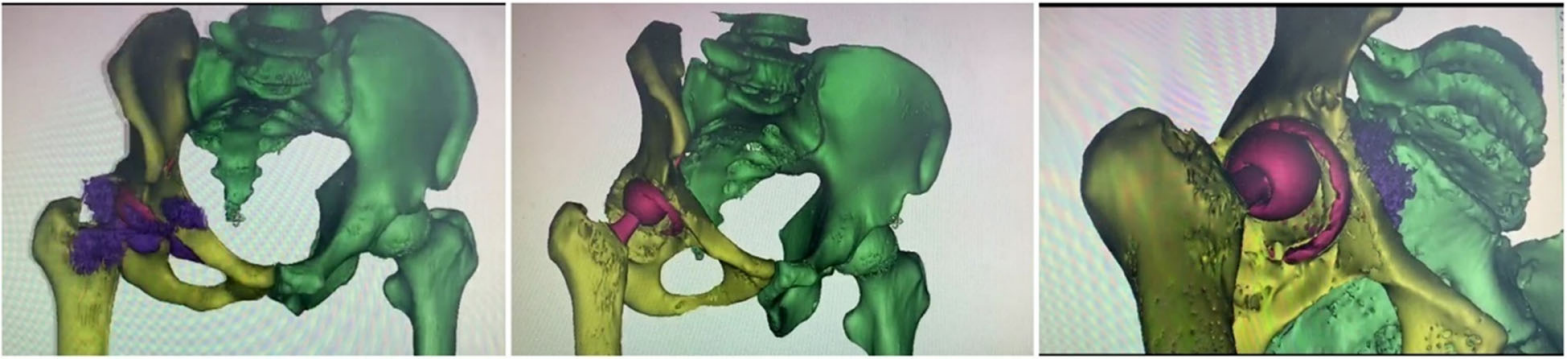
3D computed tomography reconstruction revealed numerous metal debris (purple) around hip joint and worn of the ceramic head and acetabular shell
